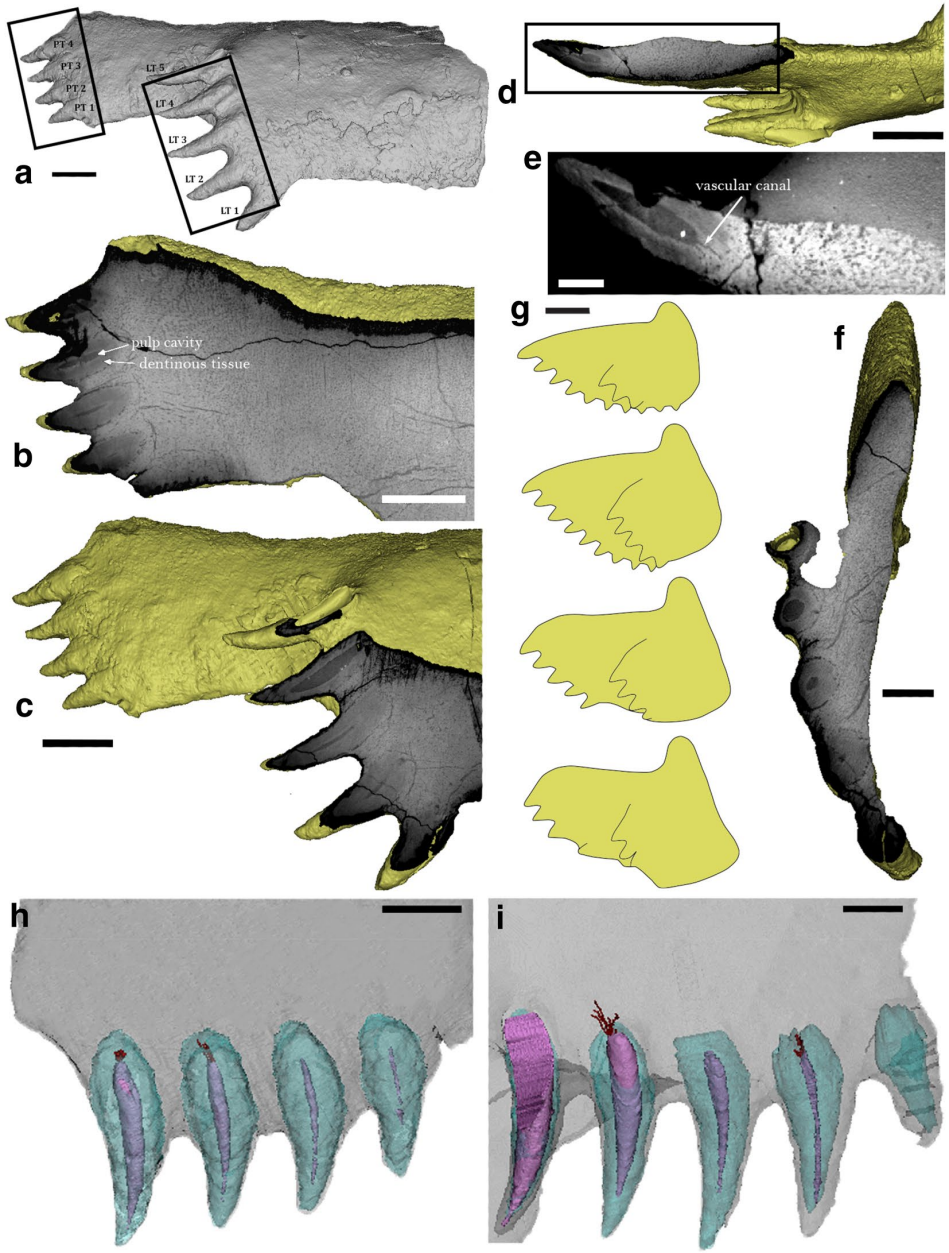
CT slices of the posterior superognathal of *Leptodontichthys ziregensis* gen. et sp. nov. (PIMUZ A/I 5022). a Overview in labial view. b–f, both tooth rows showing layers of dentin and the pulp cavities. b Parasagittal view of the posterior row and c of the lateral row. d, e, transverse sections (e: close-up). f Frontal section. g Growth development of *Plourdosteus canadensis* according to Ørvig (1980). h Posterior row and i lateral row. Both tooth rows of *L. ziregensis* gen. et sp. nov. were 3D reconstructed to show these layers and some preserved basal canals. Scale bars represent 10 mm (a–d, g), 2 mm (e), 4 mm (f) and 5 mm (h, i). LT, Lateral Tooth; PT, Posterior Tooth
